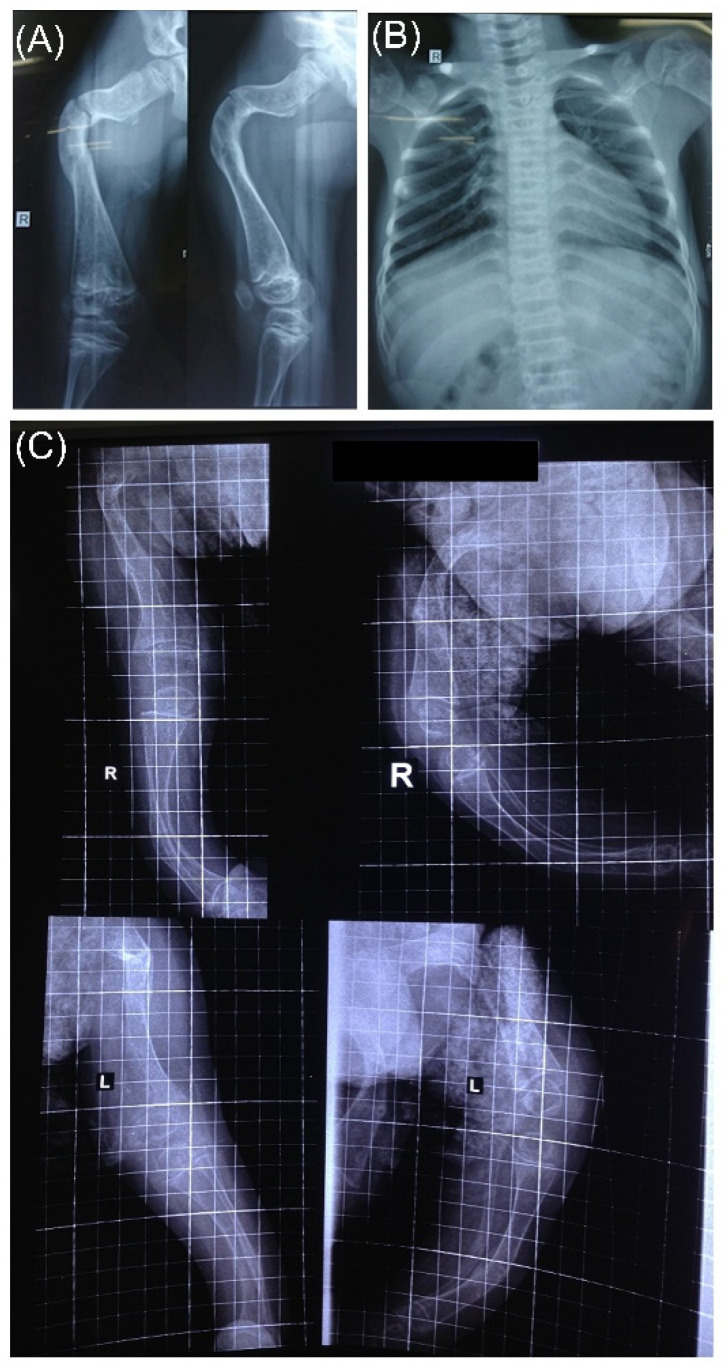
Radiographs of patients VN11 and VN75. (A) Radiograph of right lower limb of patient VN11; (B) chest radiograph of patient VN11; (C) radiograph of lower limbs of patients VN75, R, indicates right; L, indicates left lower limbs.
